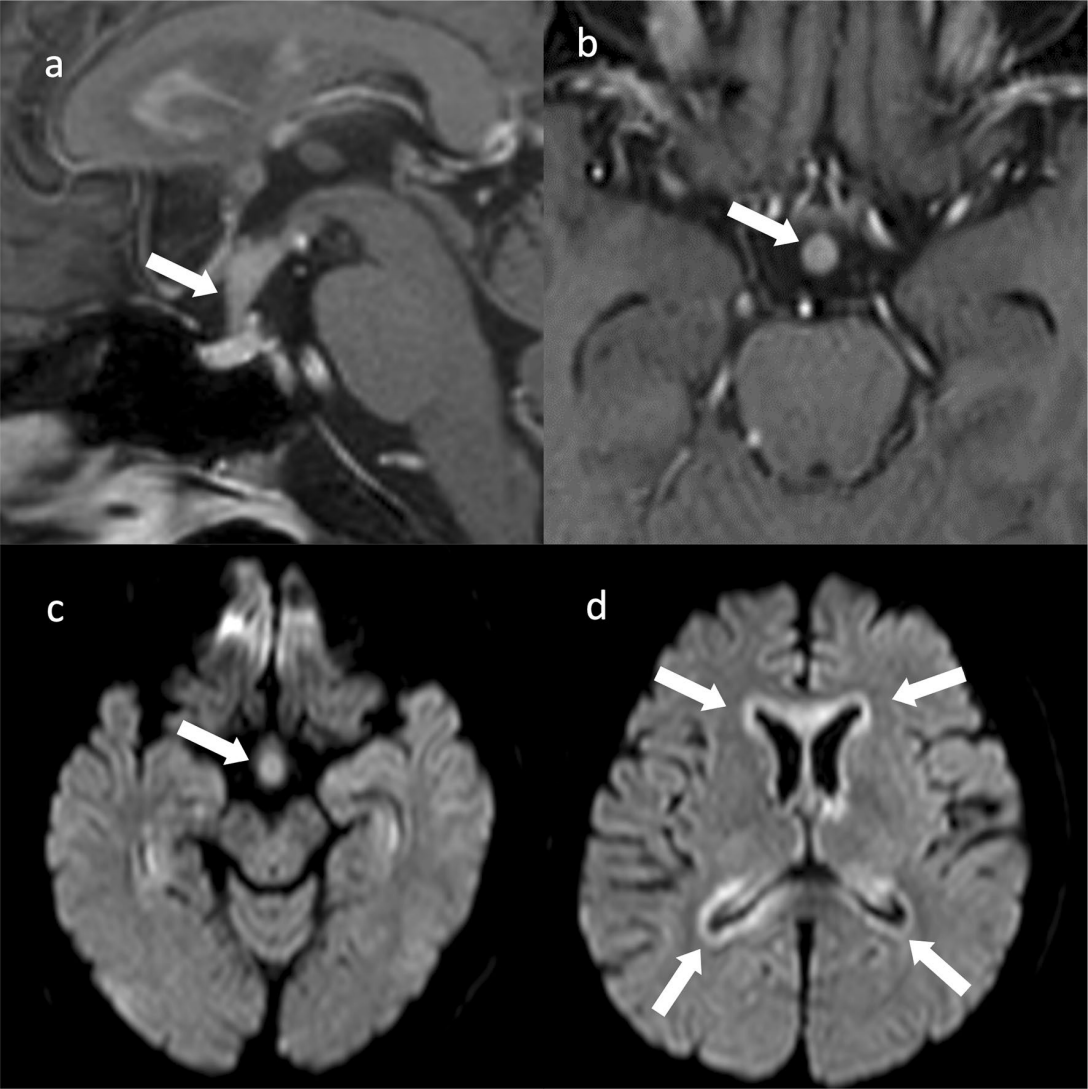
Primary central nervous system lymphoma (PCNSL) involving the pituitary stalk. A 60-year-old male with generalized body malaise. The pituitary stalk is enlarged with contrast enhancement on contrast-enhanced T1WI (arrows) (a, b) and hyperintensity on diffusion-weighted imaging (DWI) (arrow) (c). d DWI showing hyperintensity lesion along the ventricular wall DWI showing hyperintensities along the ventricular wall (arrows), which represents hypercellularity. Brain biopsy confirmed a diffuse large B-cell lymphoma
